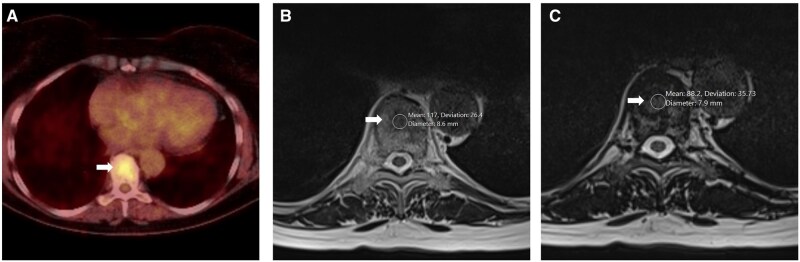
T9 vertebral body lesion (white arrow) in a 76-year-old female undergoing investigation for a solitary lung lesion. (A) Axial FDG PET imaging demonstrates a focal avid lesion with SUV of 4.6, stable on follow up FDG PET 2 years later (A). (B, C) Axial MRI Dixon sequences demonstrated greater than 20% signal drop-out with mean signal intensities of 117 in-phase (B) vs 88.2 out-phase (C), suggestive of fat content.
